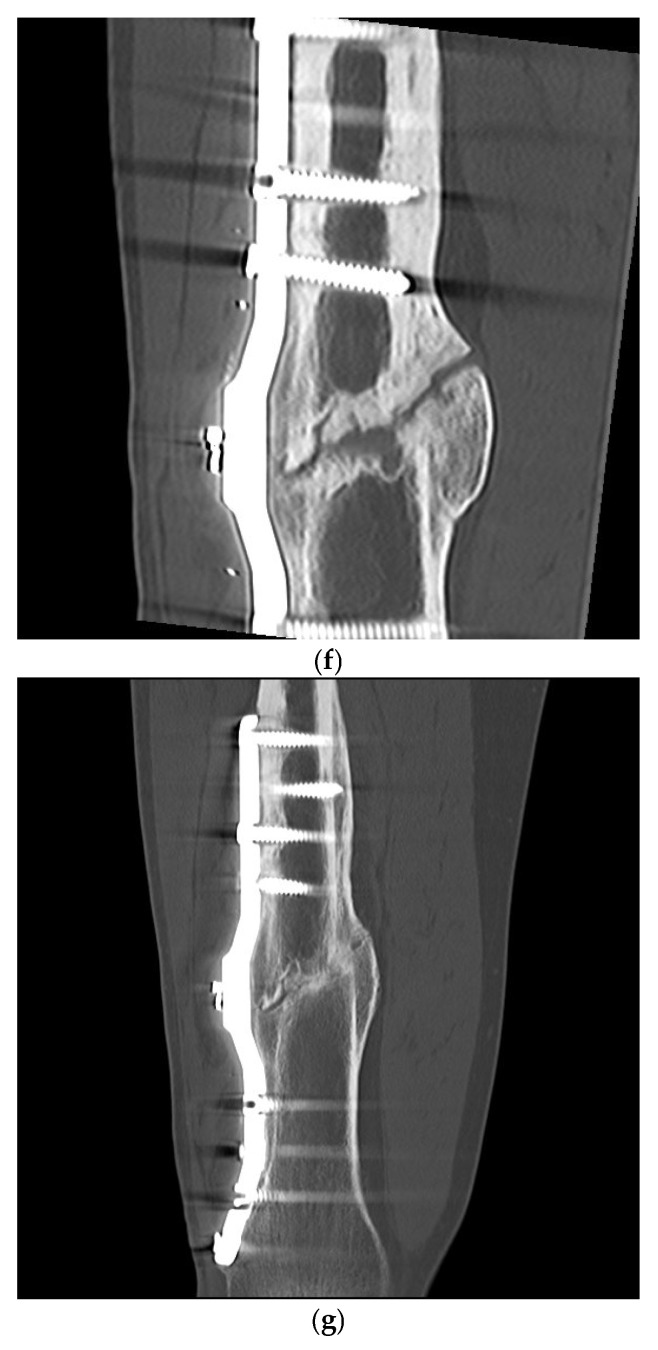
Computed tomography examinations over the course of time, preoperative (a), postoperative situation after 26 and 38 weeks (b,c), the situation after 1, 1.5 and 3.5 years (d–f), and the last result after 10.5 years (g). A coronal reconstruction is shown.
